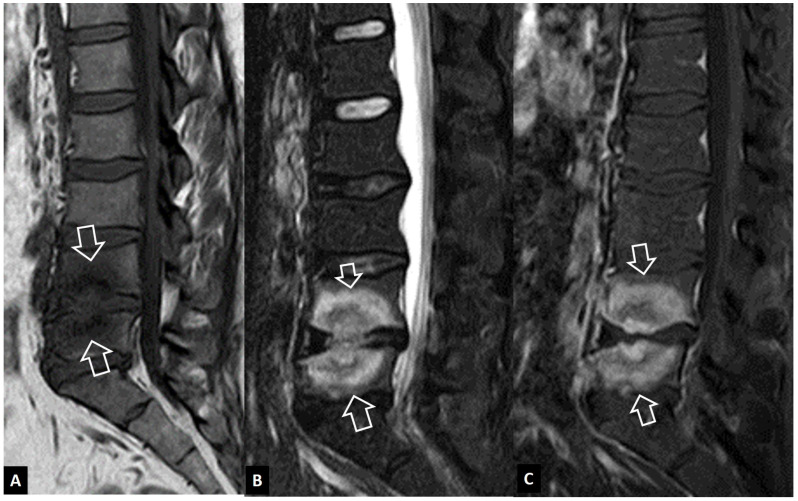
Aseptic spondylodiscitis (Andersson’s lesion) in a 38-year-old male patient with a history of 7-year low back pain. (A) Sagittal T1-w MR image showing low signal intensity at the endplates of the L4–L5 disc space (arrows). (B) Sagittal STIR MR image showing the bone marrow edema with hemispheric configuration (arrows). (C) Sagittal fat-suppressed contrast-enhanced T1-w MR image showing enhancement of the bone marrow edema (arrows) but no enhancement in the disc.
